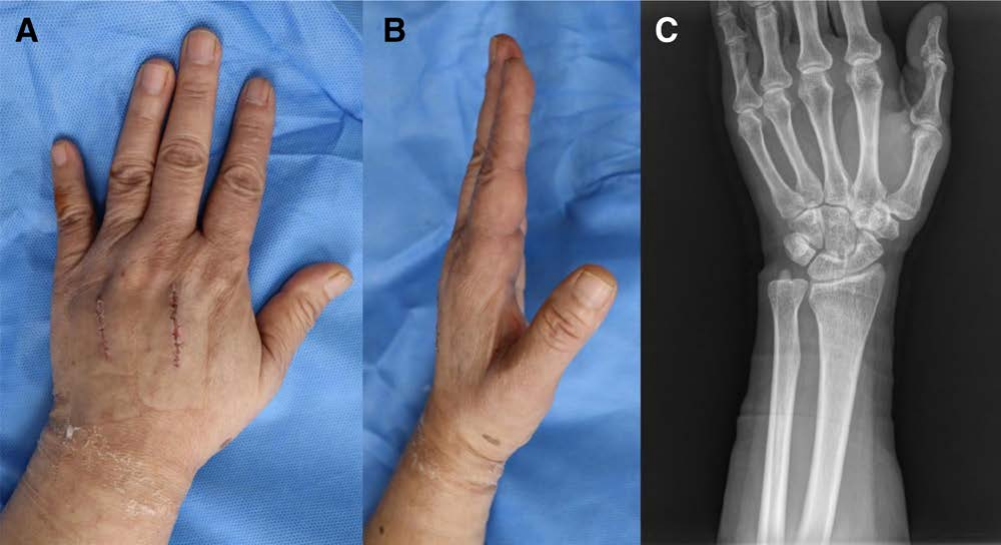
Final outcome on postoperative day 18. (A) Dorsal view of the left hand after suture removal showing well-healed incisions with minimal scarring. (B) Lateral view demonstrating full soft tissue recovery and restoration of contour. (C) Follow-up radiograph showing a complete resolution of soft tissue edema and absence of structural complications.
